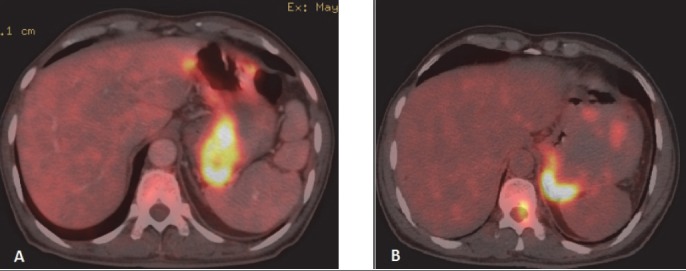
NHL of stomach. FDG avid plaque like thickening of wall of stomach is seen along the greater curvature of the stomach (A). Repeat scan during post therapy response assessment shows partial metabolic response (B)
